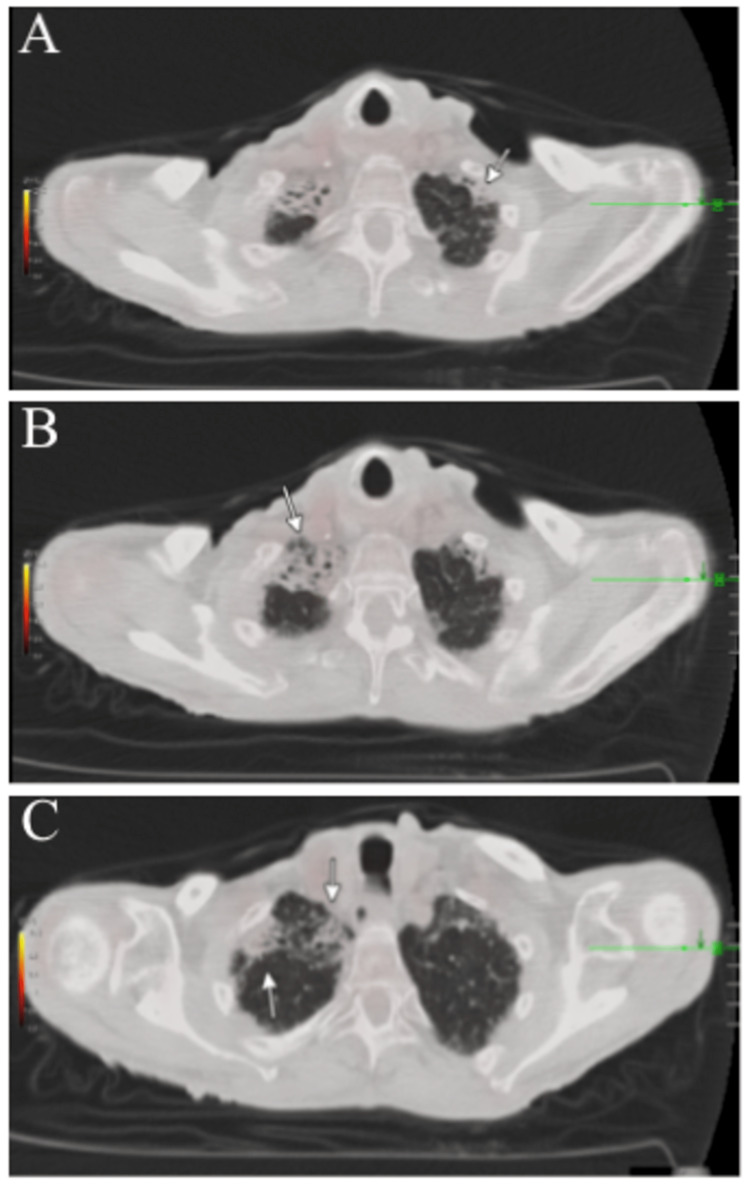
Positron emission tomography CT skull base to mid-thigh; coronal section demonstrating pulmonary fibrotic changes in the lungs CT: computed tomography
